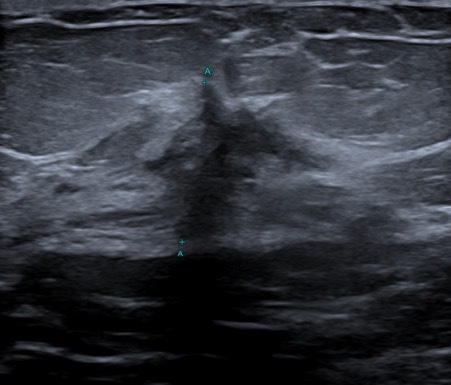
Right breast ultrasound of the spiculated lesion identified on the mammogram.
